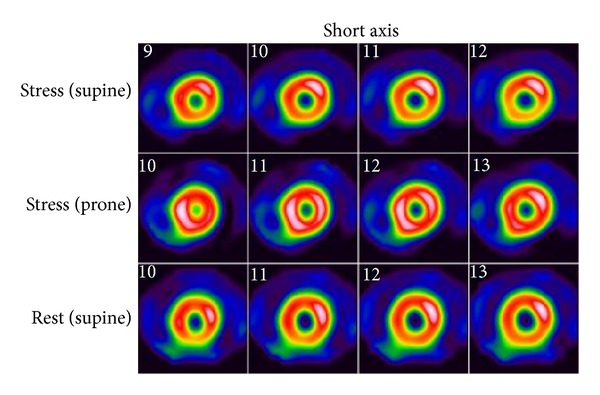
^99m^Tc-tetrofosmin rest/stress SPECT MPI study from the University of Ottawa Heart Institute in a 62-year-old female with delayed prone stress images (*middle panel*). Supine only rest/stress images suggest right coronary artery ischemia. Stress prone images show uniform radiotracer distribution confirming subdiaphragmatic attenuation artifact.
